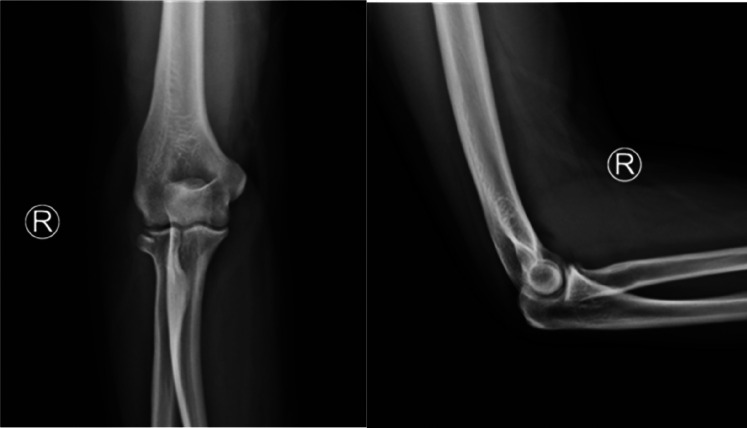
Zhang XX, male. The patient suffered from pain and limited motion of the right elbow joint after a fall. Diagnosis: Mason type II radial head fracture of the right side. Surgical method: Open reduction and screw internal fixation. Preoperative AP and lateral radiographs demonstrated significant fracture displacement.
